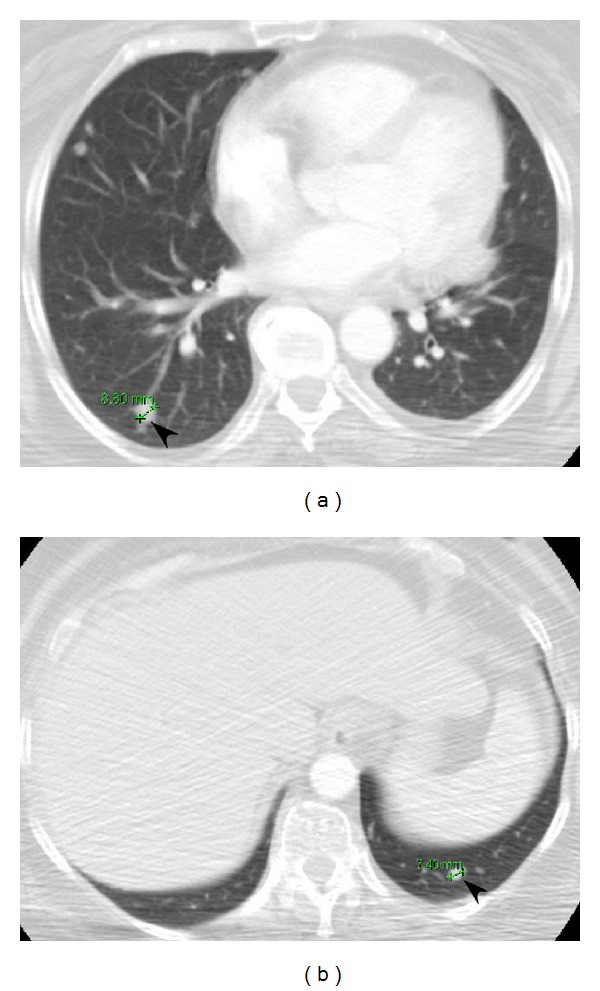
Axial CT scan images of the thorax (panels (a) and (b)) demonstrating the interval development of pulmonary metastases (arrowheads).
